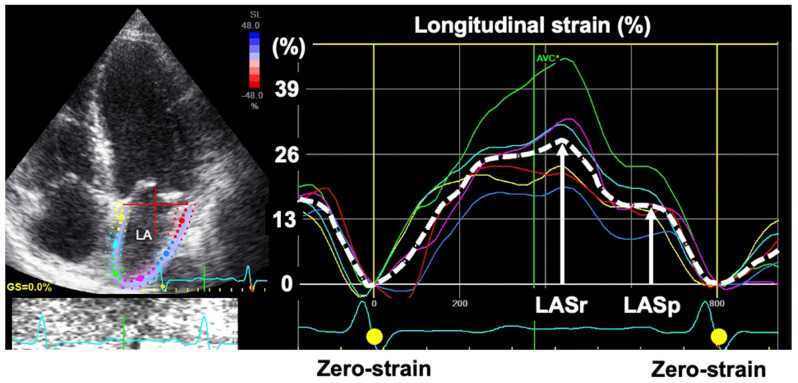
Calculation of left atrial strain. LA strain can be analyzed from a non-foreshortened apical four-chamber view. The zero-strain reference is set at end-diastole according to the recommendation by Badano et al. [17], enabling one to obtain LA strain value even in patients with atrial fibrillation. LA reservoir strain (LASr) is calculated as difference between end-diastole and onset of early LV filling, while LA pump strain (LASp) as difference between onset of atrial contraction and onset of late LV filling. LA, left atrial, LV, left ventricular.
